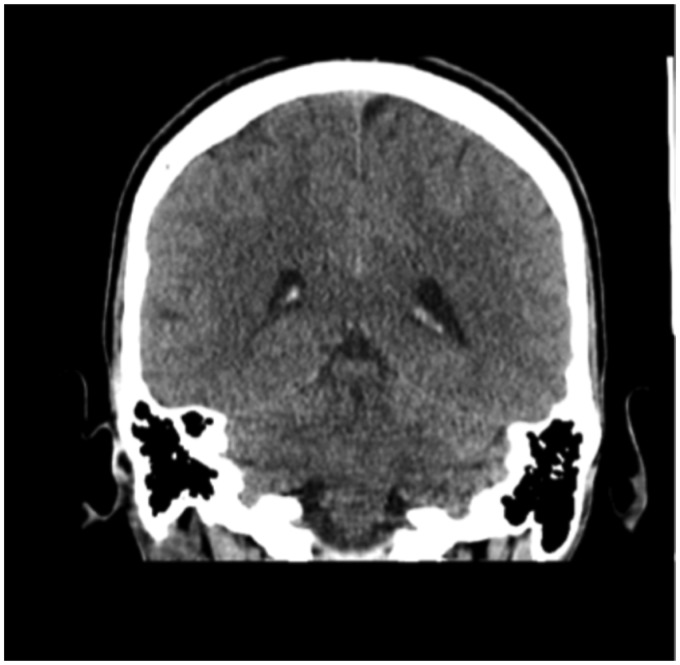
CT brain showing normal appearances.
